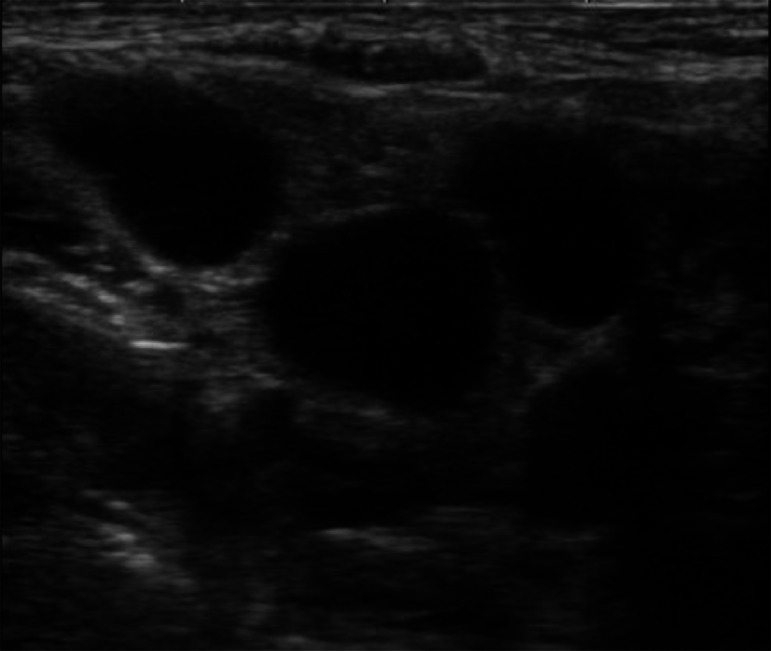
Ultrasound (US) findings. US showed enlarged oval lymph nodes with very low internal echoes in the right supraclavicular region.
